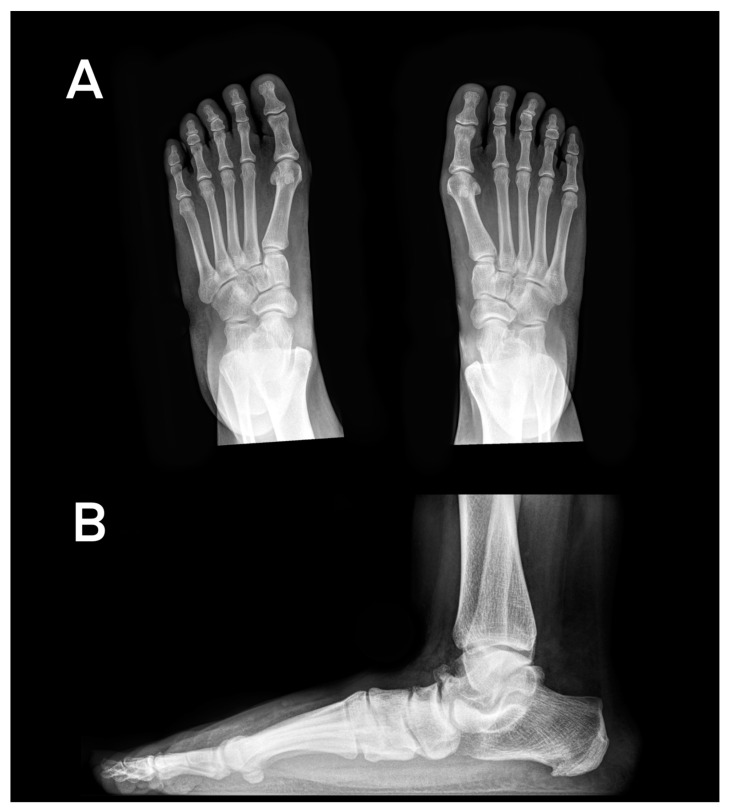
(A): Weight-bearing, dorsoplantar radiographs of the feet presenting with hallux valgus (HVA > 15°), elevated TCA, and mild JSN of the talonavicular joint. (B): Lateral radiograph of the left foot and ankle presenting with moderate JSN of the TN joint, mild JSN of the CN joint, and a decreased Meary angle and calcaneal inclination angle.
